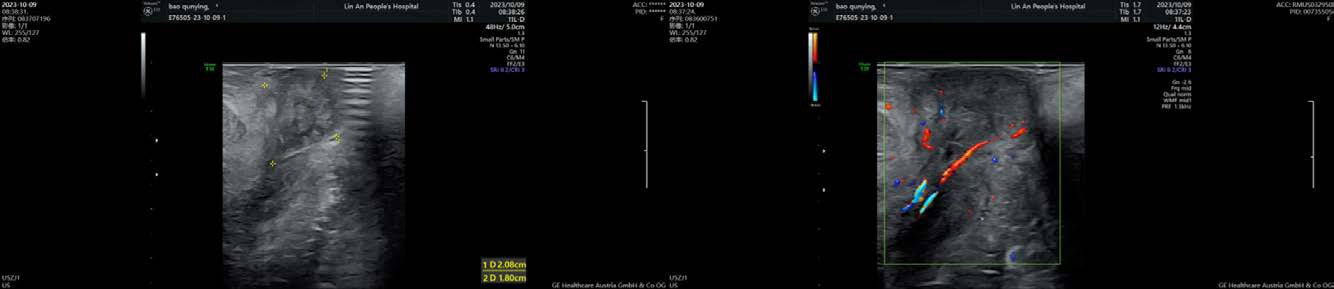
3D pelvic ultrasound examination indicated that the tumor originated from the mid-urethra with vascularity at the base.
